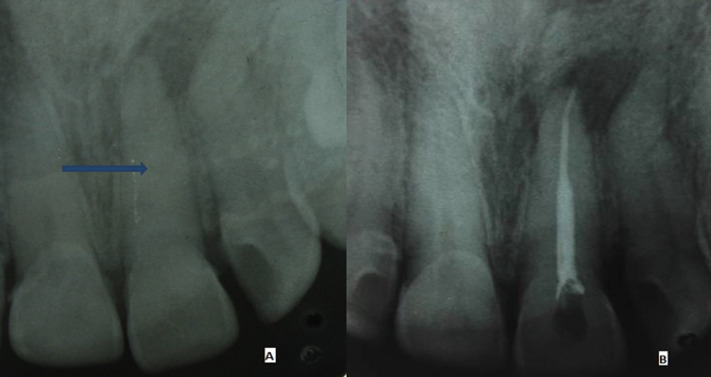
periapical radiographs of maxillary right central incisor: A) preoperative: calcified canal (blue arrow); B) obturation
